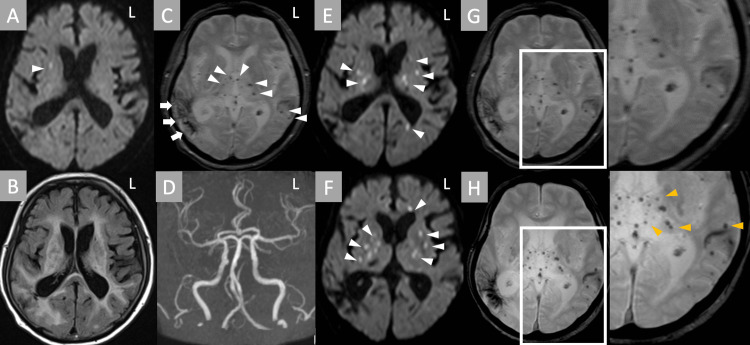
Imaging results. A: Axial diffusion-weighted imaging (DWI) showed hyperintense lesions in the right basal ganglia (arrowhead). B: Fluid attenuated inversion recovery showed broad white matter lesions and severe subcortical white matter lesions. C: T2*-weighted imaging (T2*WI) showed multiple cerebral microbleeds both in the thalamus and subcortical region (arrowheads), in addition to the pre-existing intracerebral hemorrhage (arrows). D: MRA showed no occlusion or stenosis in the major cerebral arteries. E, F: Axial DWI showed multiple hyperintense lesions in the bilateral small vessel territory (arrowheads). G, H: Compared with initial T2*WI (G), cerebral microbleeds became more pronounced in the follow-up MRI (H) (arrowheads). L, left; MRA, magnetic resonance angiography.
